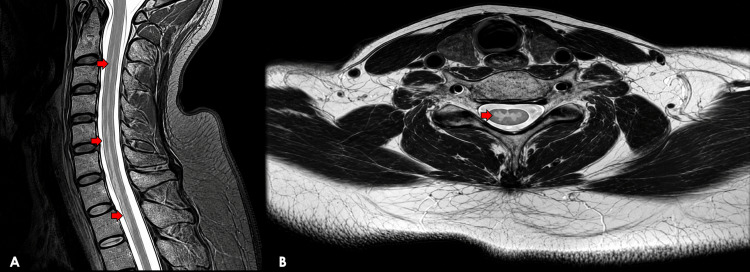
Cervicothoracic spine MRI demonstrating a longitudinally extensive intramedullary myelopathy. (A) Sagittal T2-weighted image demonstrates a poorly marginated, non-expansile T2-hyperintense intramedullary lesion extending contiguously from C3 to T2 (red arrows). (B) Axial T2-weighted image demonstrates predominant central intramedullary involvement without significant spinal cord enlargement, focal mass effect, or compressive pathology (red arrow). Diffusion-weighted imaging demonstrated no restricted diffusion, and no discrete intramedullary enhancement was identified on post-contrast imaging.
